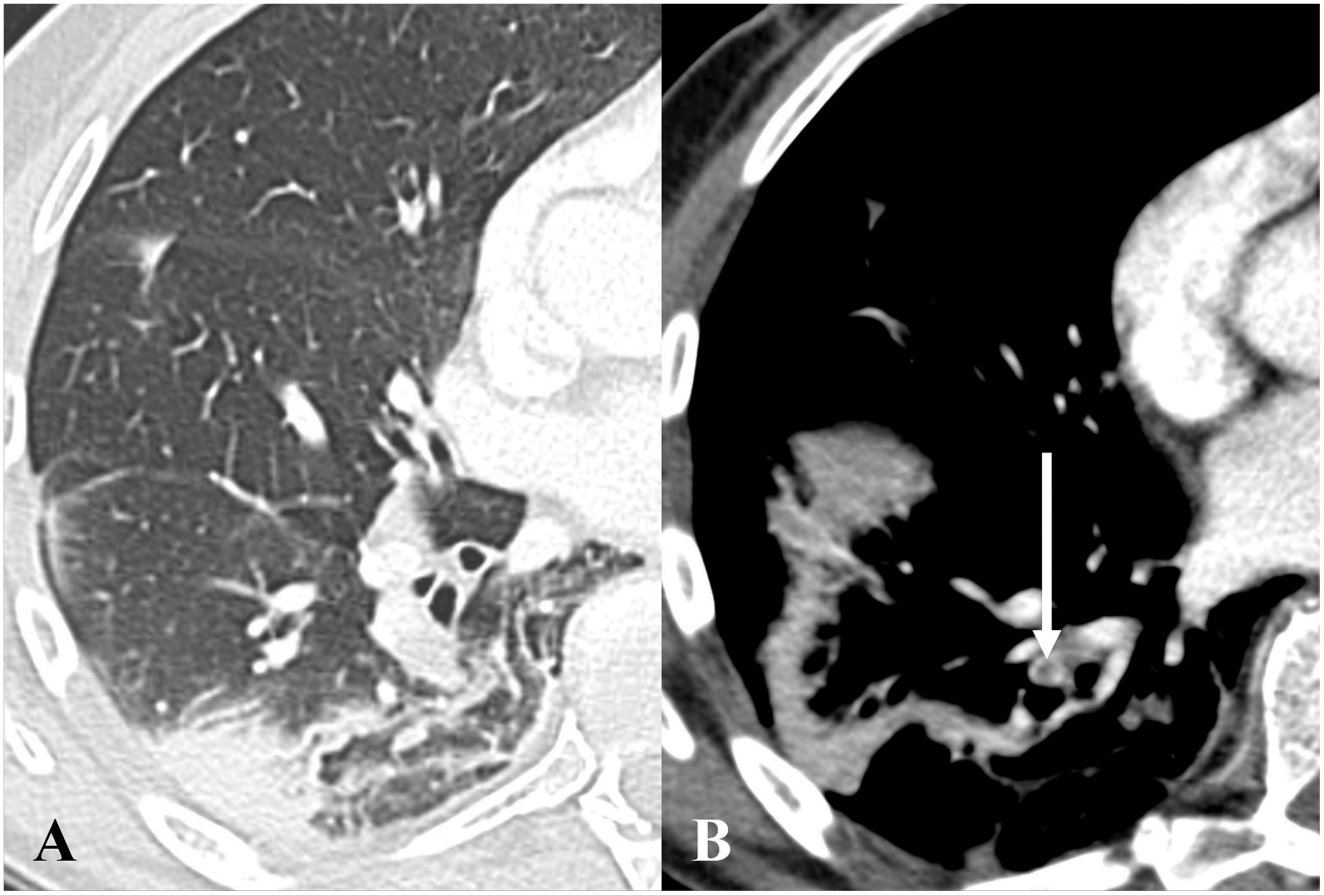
Axial CT pulmonary angiography in lung (A) and soft tissue windows (B), in a 68-year-old male patient presenting with bilateral acute pulmonary embolism and associated basal atelectasis and pulmonary infarction. One of multiple emboli is depicted on (B) (white arrow). The radiology technician scored the Qanadli obstruction index (QOI) 35% with intermediate risk-stratification, while the radiologist scored the QOI 40% with high risk-stratification.
